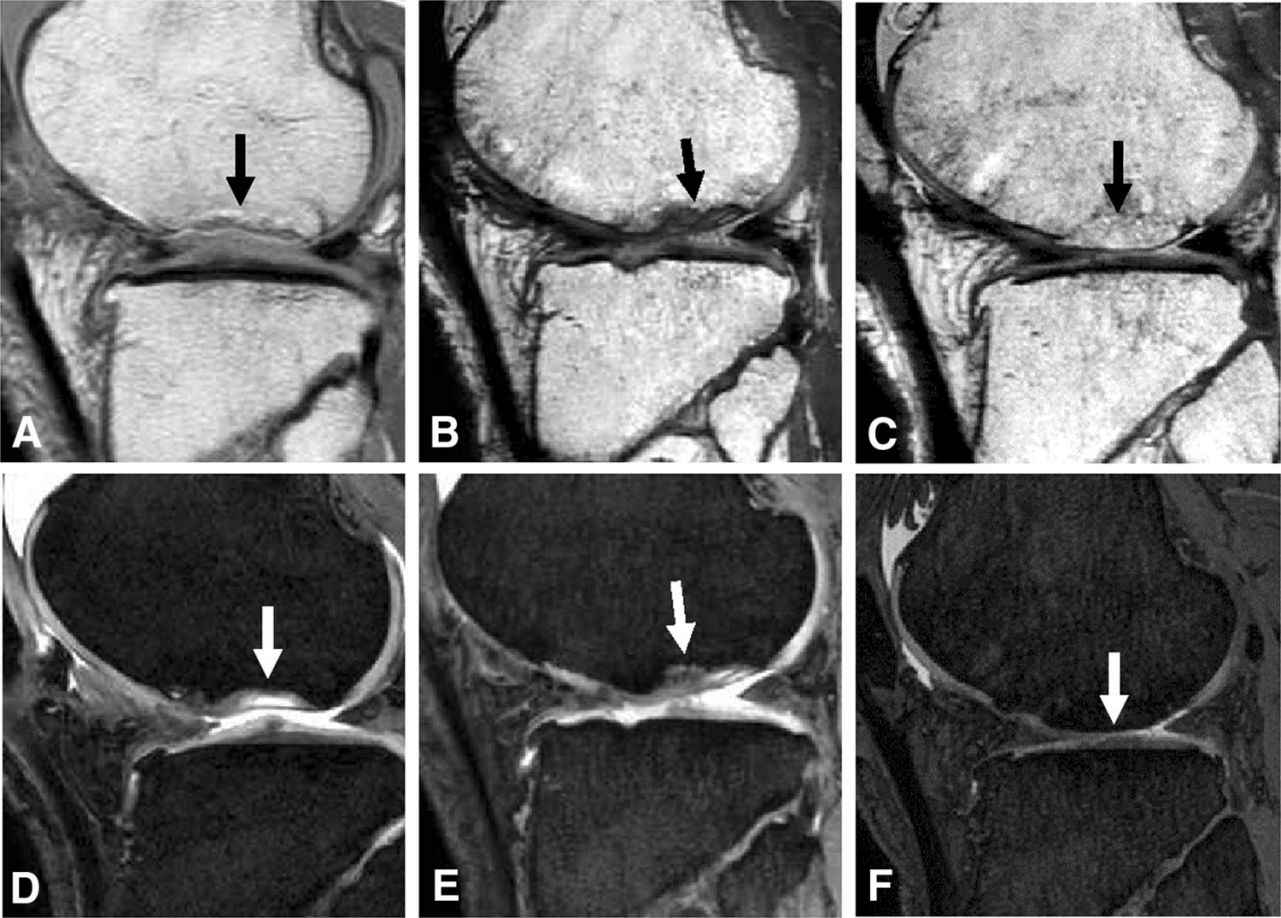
Sequential MRI features of Patient 2 who had an osteochondral defect, indicated by the arrow, are shown (A) for bone before treatment, (B) at 6 months, and (C) at 6 years, and for (D) cartilage before treatment, (E) at 6 months, and (F) at 6 years. The sequential MR images showed that the osteochondral defect was filled with cartilaginous tissue at 6 months. The bone defect was filled with bony tissue, and the cartilage defect was incompletely filled with cartilaginous tissue at 6 years.
